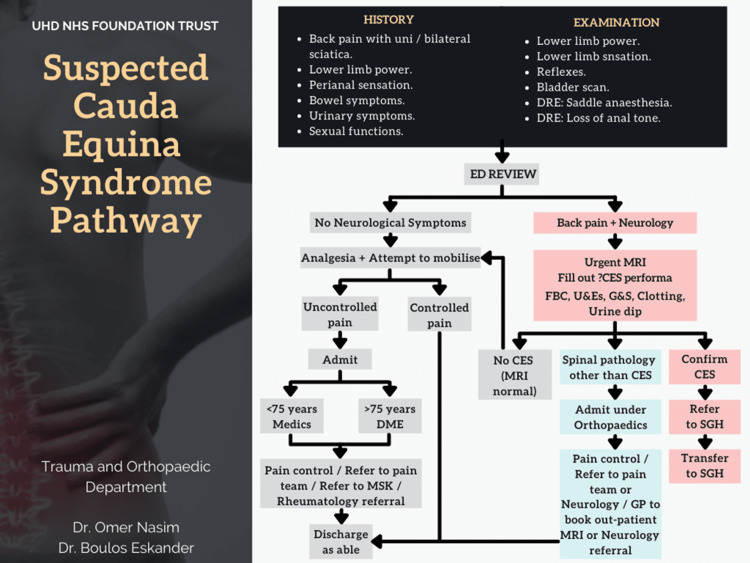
The proposed pathway for patients suspected with CES (1st PDSA cycle) CES: Cauda equina syndrome, PDSA: Plan-do-study-act, DRE: Digital rectal examination, ED: Emergency department, MRI: Magnetic resonance imaging, DME: Department of medicine for the elderly, SGH: Southampton General Hospital (tertiary care hospital) Image designed by author Omer Nasim, and created by author Boulos Eskander
